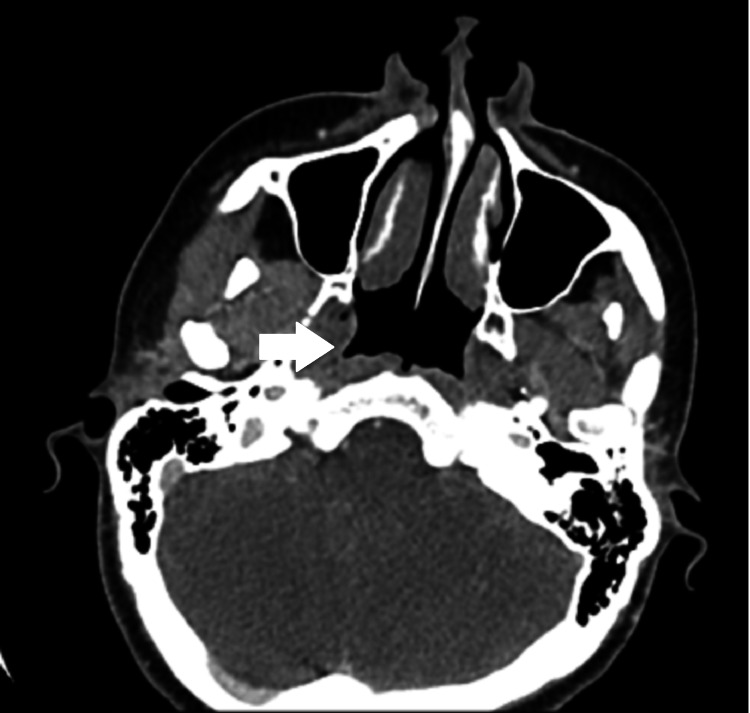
Axial CT image of the nasopharynx An axial CT image of the nasopharynx shows no lesion. The Fossa of Rosenmuller is preserved.
